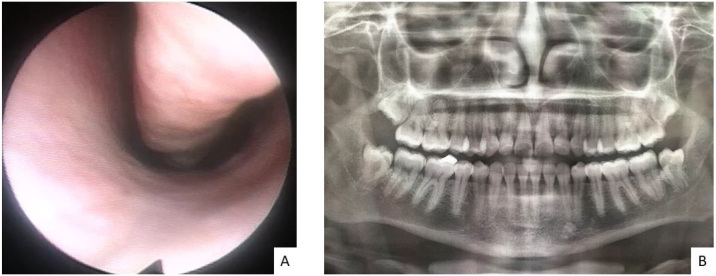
Complementary exams. (A) Flexible nasolaryngoscopy shows the inferior meatus of the left nostril. No signs of fistula on the nasal floor. (B) Panoramic radiograph of the face without bone erosion.
